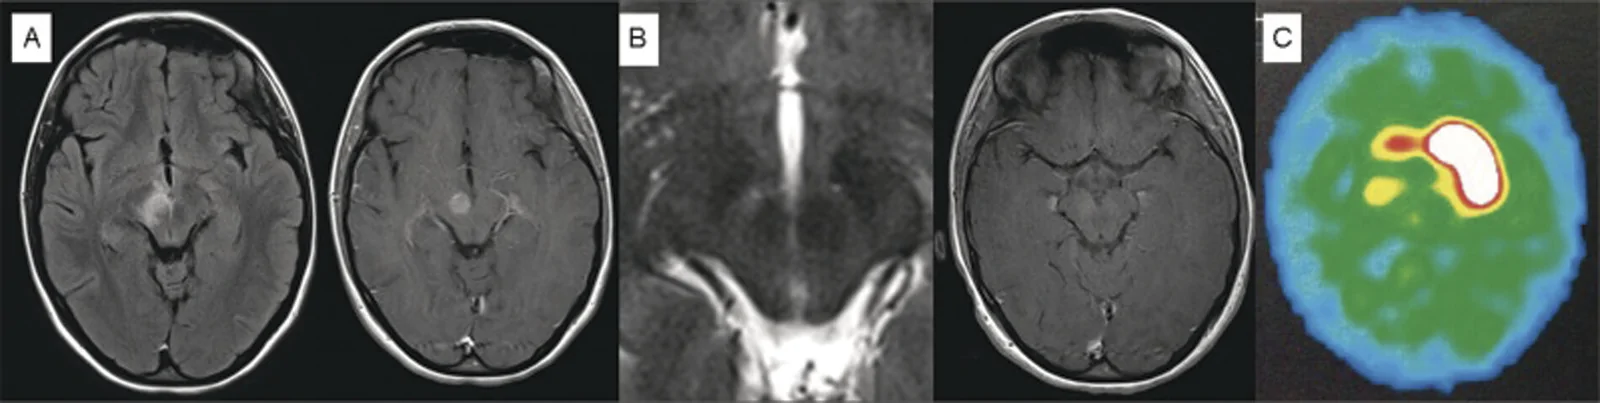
Holmes' tremor caused by an inflammatory demyelinating lesion. MRI findings. (
